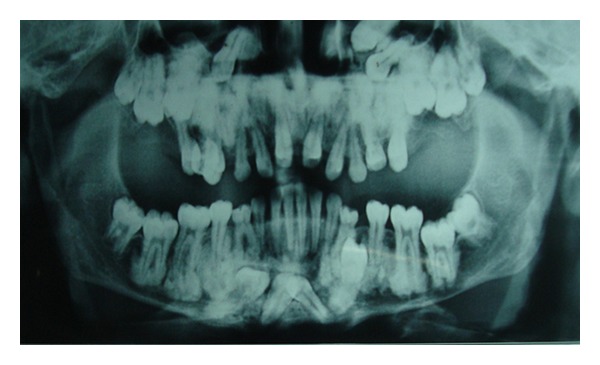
Panoramic radiograph (Case 1).
